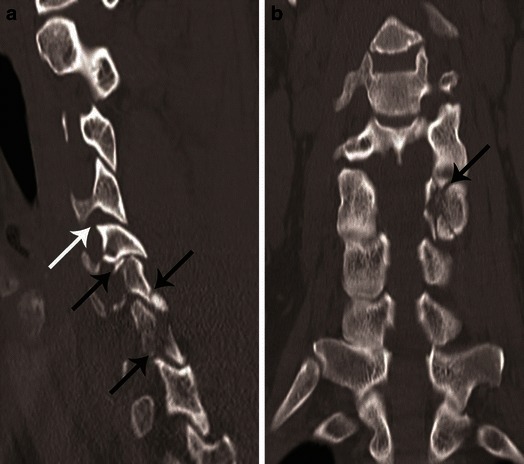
Hyperextension compression injury. a Parasagittal CT image shows C6, C7 articular pillar and process comminuted fracture with vertical orientation (*black arrows*). Focal anterior widening of C4–C5 facet joint (*white arrow*) is a characteristic feature of hyperextension injury. b Coronal CT image from the articular pillar in a different patient shows left C5 articular pillar fracture with vertical orientation and comminution (*black arrow*)
